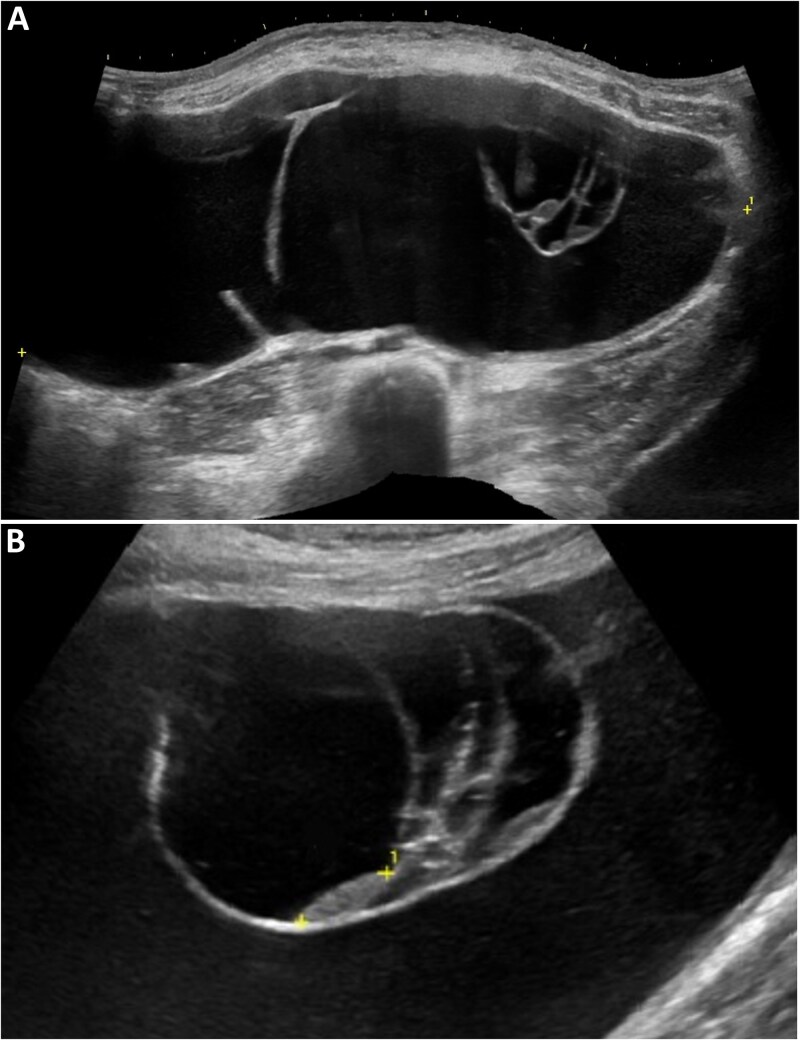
Transabdominal ultrasound. (A) Panoramic transabdominal ultrasound demonstrating a giant multiloculated cystic mass occupying the abdominopelvic cavity, with predominantly anechoic content. (B) Detailed view showing internal septations, some appearing thickened, without definite solid nodules or vascularized components.
